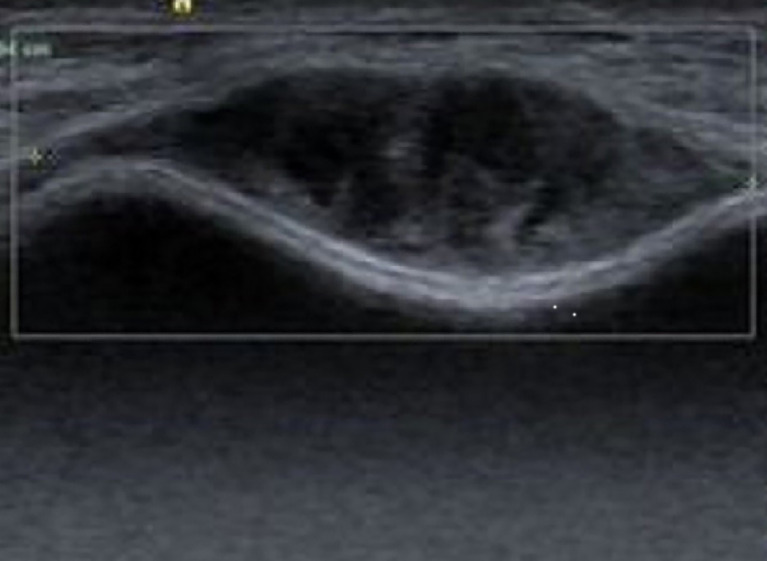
A regular hypoechoic mass on breast ultrasonography.
